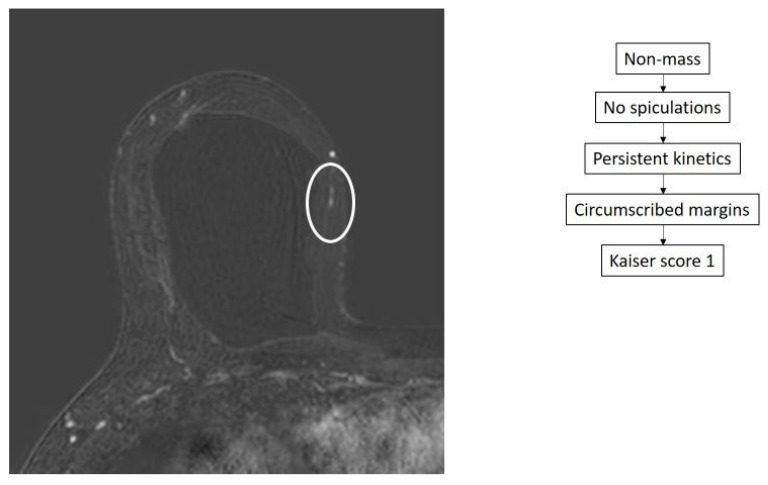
False negative case of a 55-year-old woman with recently diagnosed ductal carcinoma in situ (DCIS) in the lower outer quadrant of the right breast. An additional tiny linear non-mass enhancement is identified in the lower inner quadrant of the ipsilateral breast (circle), showing a Kaiser score of 1. MRI-guided biopsy was performed yielding DCIS.
